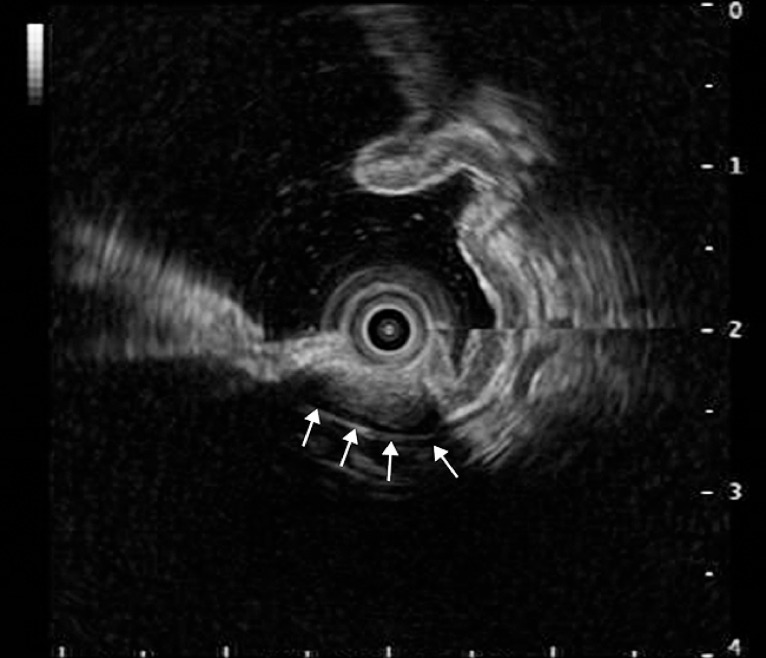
Endoscopic ultrasonography showed the hypoechoic tumor with a clear boundary and an endoluminal growth pattern, which originated from the muscularis propria (arrows).
